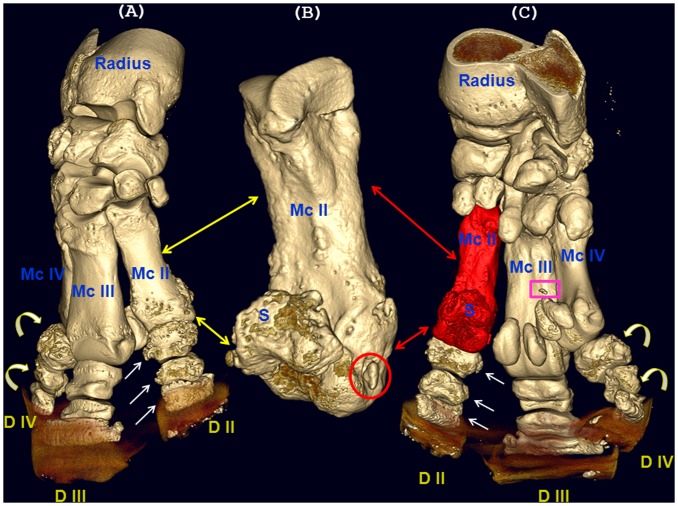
Bone pathology of the distal right front limb in rhinoceros 2. Computed tomographic tridimensional (A–C) images: (A) autopodium, medio-dorsal aspect; (B) second metacarpal bone, latero-palmar aspect; (C) autopodium, latero-palmar aspect. Second metacarpal bone (Mc II) exhibits amorphous periosteal reaction adjunct to severe remodelling of the distal epiphysis and metaphysis, associated with underlying cortical lysis prolonged in a proximal direction to the mid-diaphysis, where the cortex is irregularly thinned (long, double headed arrows). Cortical bone proliferation, periosteal reaction, and severe remodelling are also present on the neighbouring sesamoidal (S) bones (fused, with bridging bony spurs) and phalanges (Ph) I, II, III of the second digit (D II), (simple and double headed short arrows). The second phalanx and the paired sesamoids of the fourth digit (D IV) express moderate cortical remodelling, in contrast with the first phalanx where the cortical lysis (mainly dorsal) and bone remodelling are extensive, accompanied by multiple, bony fragments (curved arrows). Mineralized body (C-rectangle) and bone fragments (B-circle) are evident.
